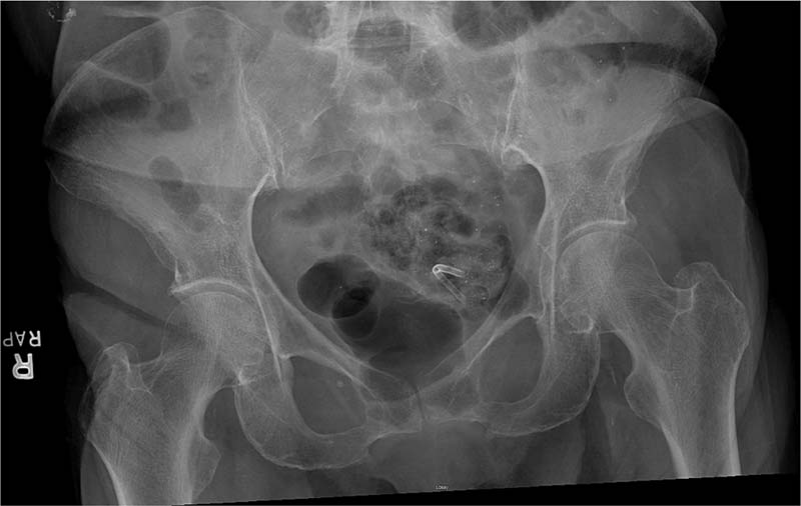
Abdominal X-ray of plastic biliary stent overlying the pelvis.
